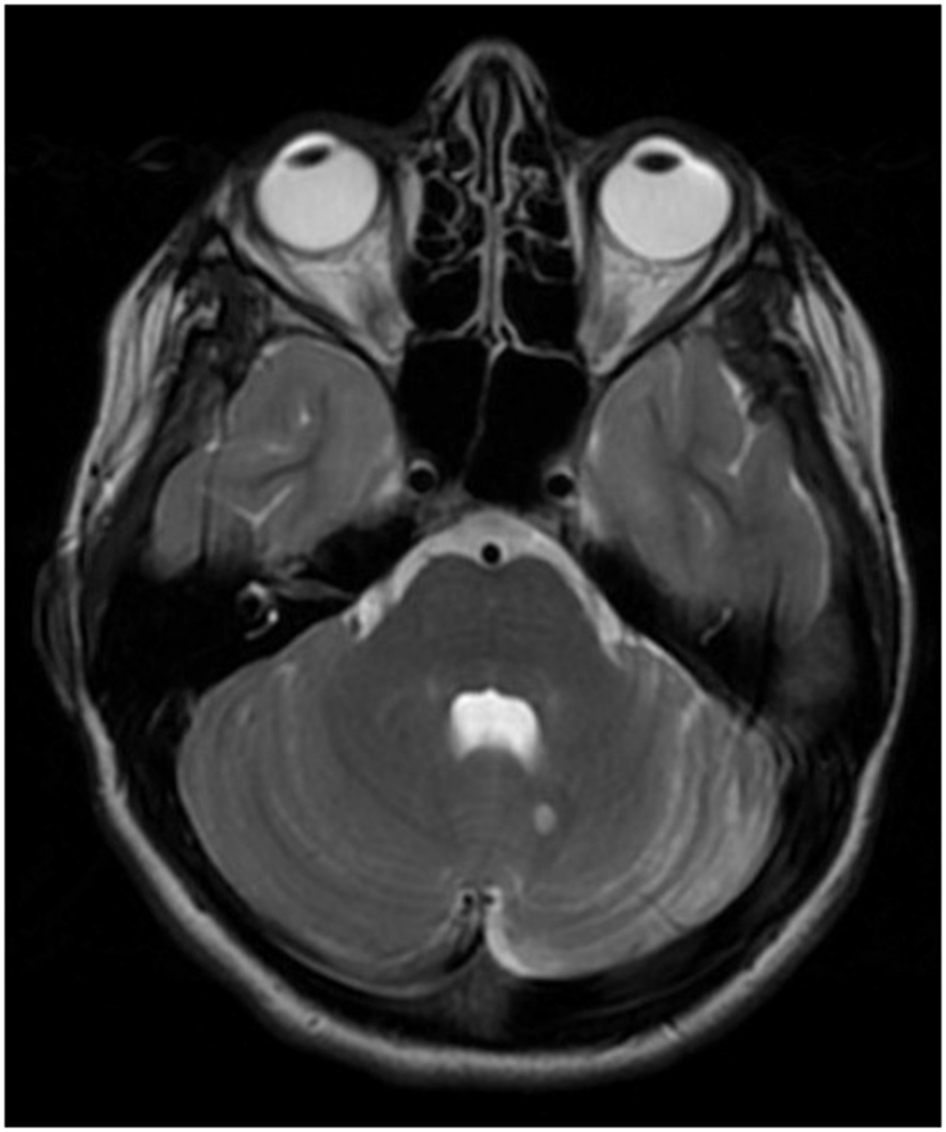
T2 sequence of brain MRI demonstrating hemi-atrophy of the left cerebellum in addition to a 6 mm T2-hyperintense lesion near the cerebellar vermis on the left. Additional brain imaging not show any further T2/FLAIR (fluid-attenuated inversion recovery) abnormalities in the cerebellum, cerebrum or corpus callosum (sequences not shown).
